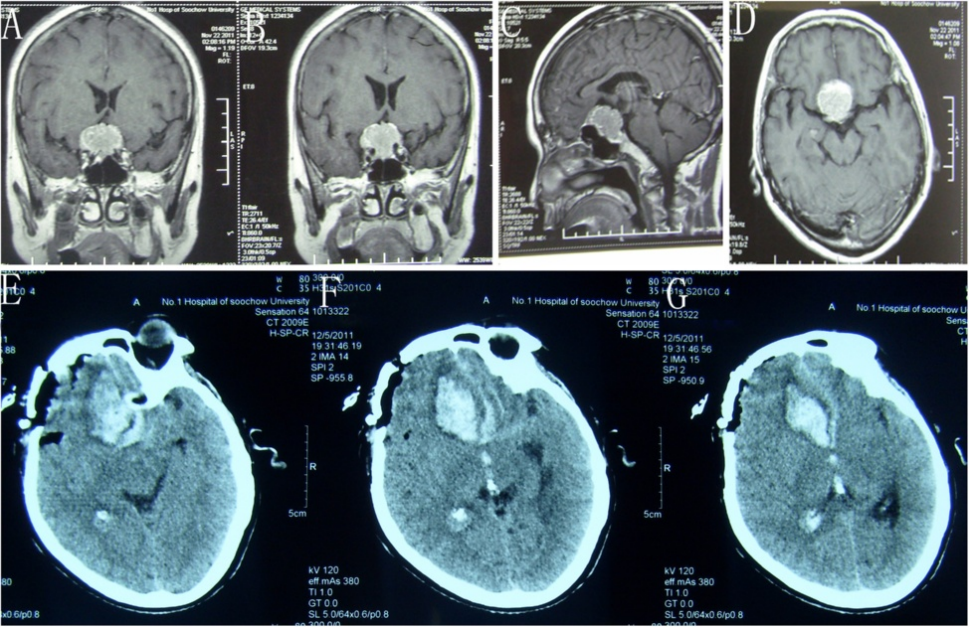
Case number 22: (A-D) view of contrasted magnetic resonance imaging showing a tuberculum sellae meningioma. (E-G) view of CT images showing a hematoma in the sellar region.
